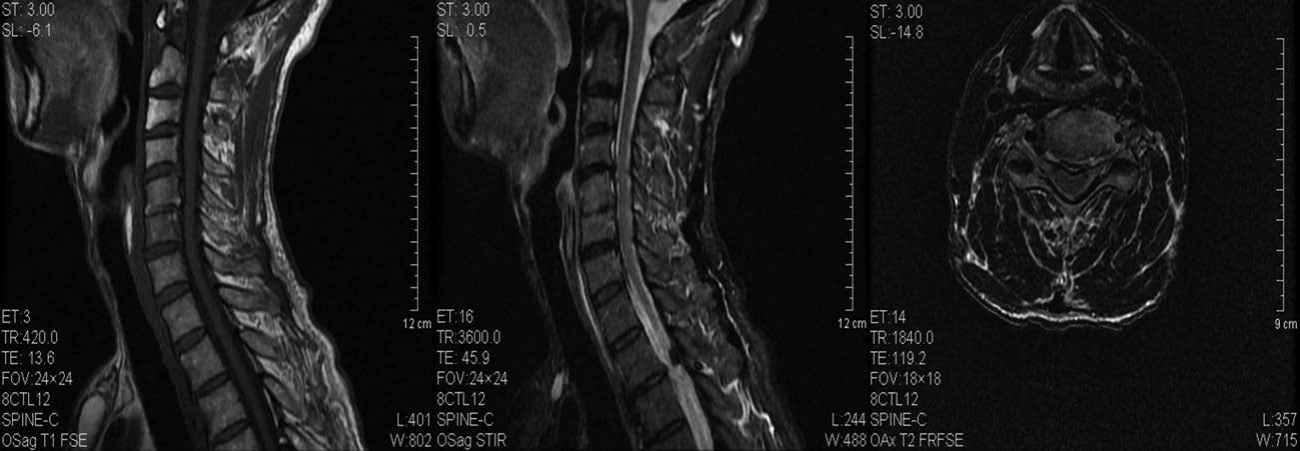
The patient cervical magnetic resonance imaging results.
